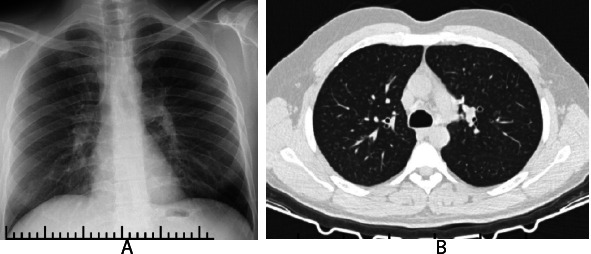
A: ILO pneumoconiosis PA lung X-ray classification p/p 0/1. B: Lung CT with small opacities.
